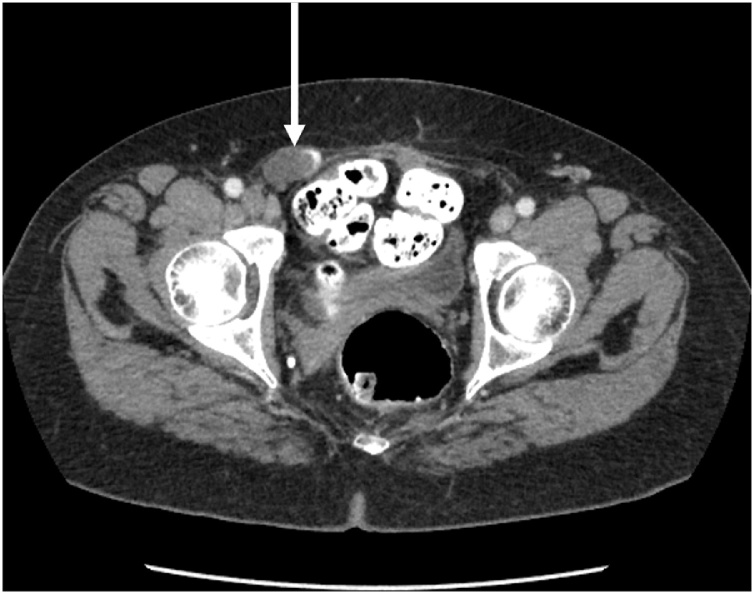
CT cut through the pelvis show a 4 cm cystic mass with peripheral calcification in the right inguinal region. This was compatible with recurrence of pseudomyxoma peritonei 6 years after initial treatment.
